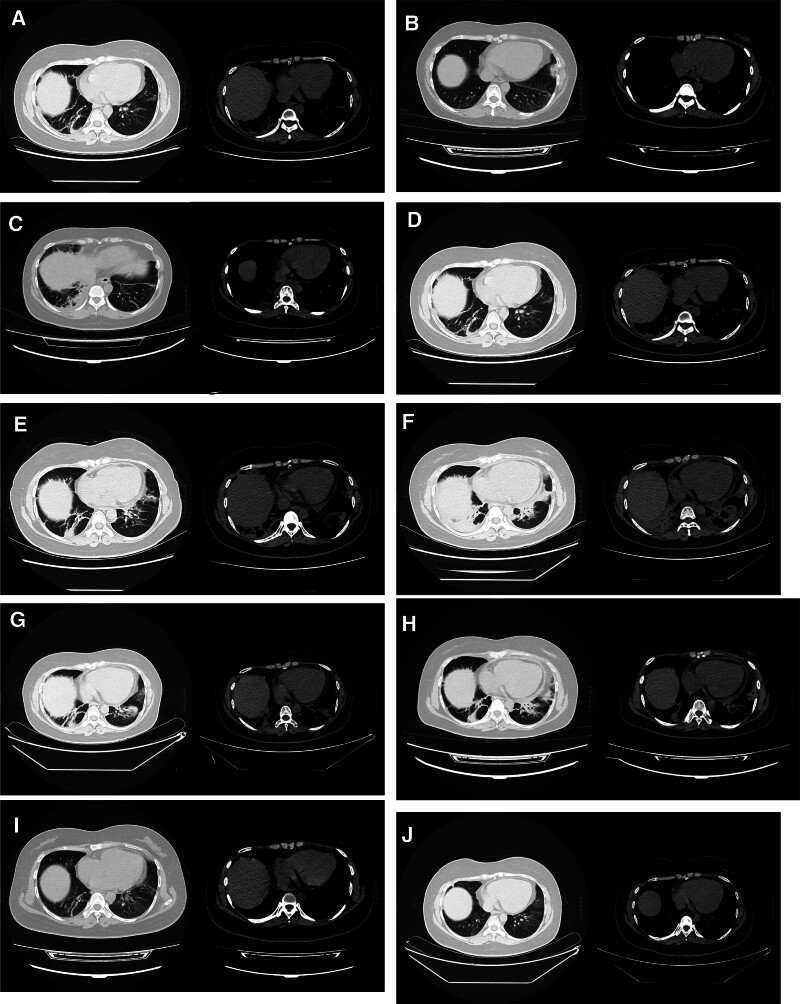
CT images of the lungs and mediastinal windows of patients at different time points. (A) Multiple patchy, streaky, dense shadows were observed in both lungs, especially in the inferior lobe. Local mild pericardial thickening, approximately 3 mm thick, was also observed. (B) 40 mg of MP was administered; both lung lesions were significantly more resorbed than before, and the TP improved. (C) MP was reduced to 6 mg QD. In addition, it was observed that the inferior lobe lesion in the right lung had progressed further compared to previous assessments, demonstrating pleural thickening. (D) MP was increased to 20 mg QD; the inferior lobe lesion of the right lung was more resorbed compared to previous assessments. (E) MP was reduced to 8 mg QD; inflammation in the inferior lobe of both lungs had progressed further compared to previous assessments. ILD was observed in the right lung, and a newly identified TP of approximately 9 mm was observed. (F) The MP dosage was not increased. The inflammation in the inferior lobes in both lungs was aggravated following anti-infective treatment compared to previous assessments, with a TP of approximately 12 mm. (G) MP was increased to 24 mg QD; the inflammation in the inferior lobe in both lungs was reduced compared to the previous resorption findings, and the TP had significantly reduced. (H) MP was reduced to 8 mg QD. Additionally, it was noted that the lesions in the inferior lobes of both lungs had increased compared to previous assessments. Furthermore, a new pleural thickening of approximately 11 mm was observed. (I) MP was not increased, and tofacitinib 5 mg BID was added. There was a notable reduction in the resorption of ILD in both lungs and a significant reduction in the TP by approximately 2 mm. (J) MP at 6 mg QD and tofacitinib at 5 mg BID were administered. There was a slight reduction in exudation due to resorption in both lungs, and no TP was observed. BID = twice daily, CT = computed tomography, ILD = interstitial lung disease, MP = methylprednisolone, TP = thickened pericardium.
